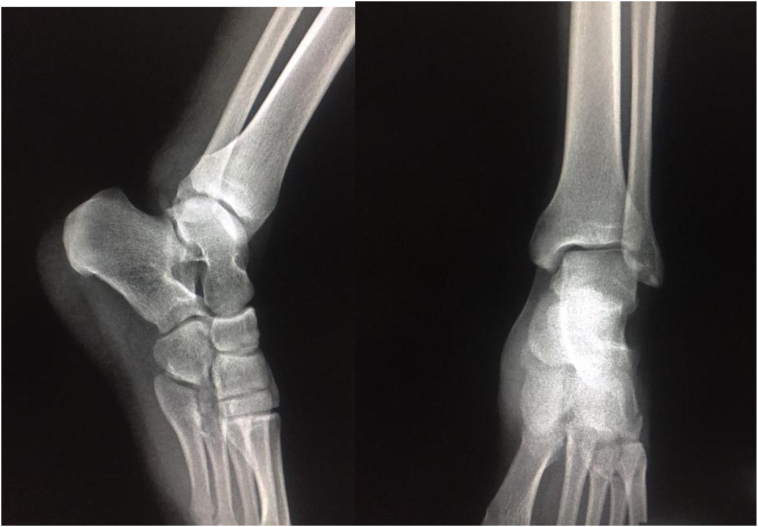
Post reduction radiograph.
